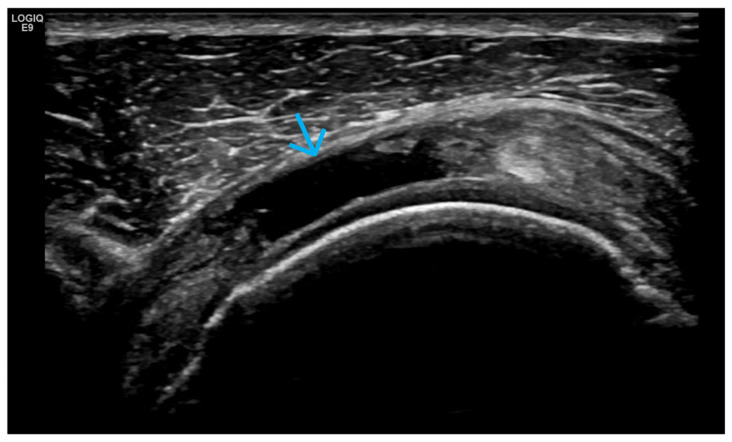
Anterior ultrasonographic scan of the shoulder joint in a patient with mechanical pain in the area due to a partial rupture of the rotator cuff. The large anechoic zone (blue arrow) corresponds to the loss of tendon fibers, resulting from their rupture.
